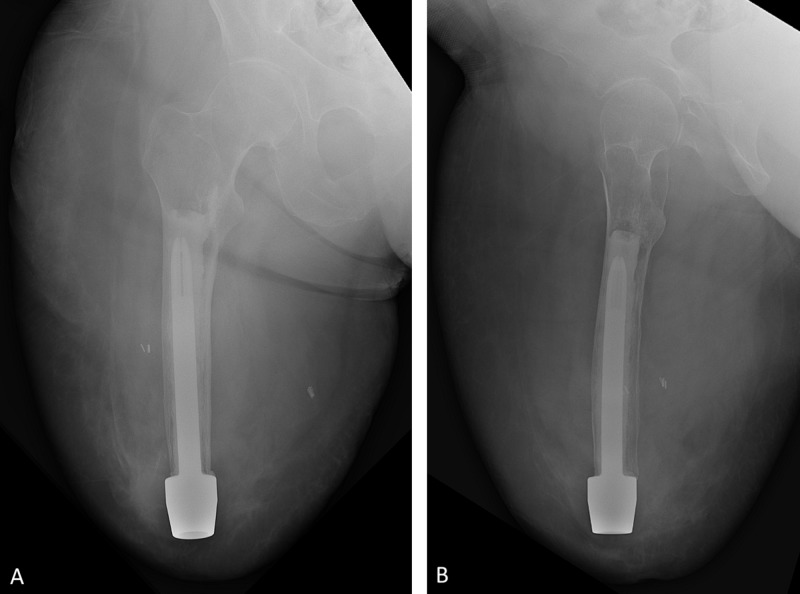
Radiographs of the right femur one month after cemented implantation. On both the anterior-posterior (A) and lateral (B) views, there is a uniform cement mantle between the implant and the cortical bone with proximal uniformity of the cement indicating the restrictor helped pressurize the cement during insertion.
